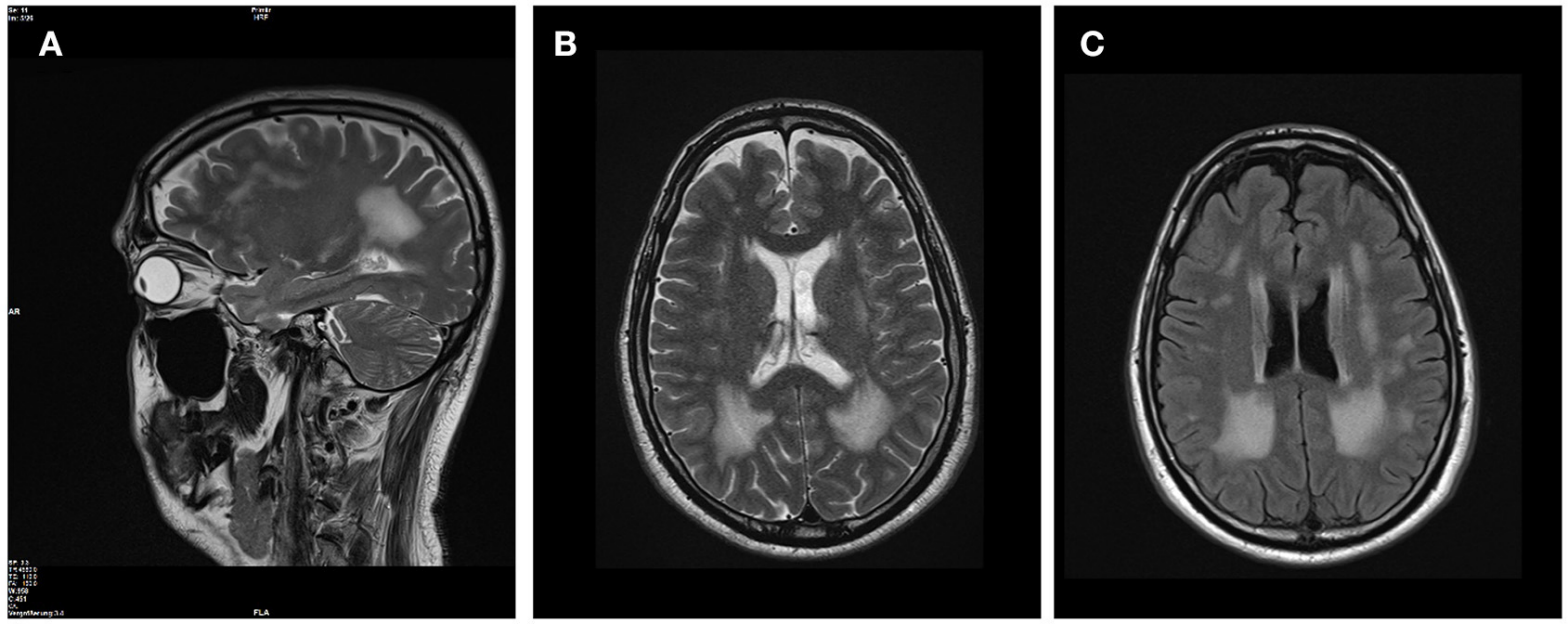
Brain MRI revealed white matter lesions typical for LAMA2-associated diseases. T2-weighted sagittal (A) and axial (B) brain MRI images revealed confluent hyperintense lesions predominantly in the periventricular white matter with involvement of the subcortical U fibers. The corpus callosum was spared, the ventricles are not enlarged and no other intracranial malformations were present (C) on FLAIR-weighted axial scan.
